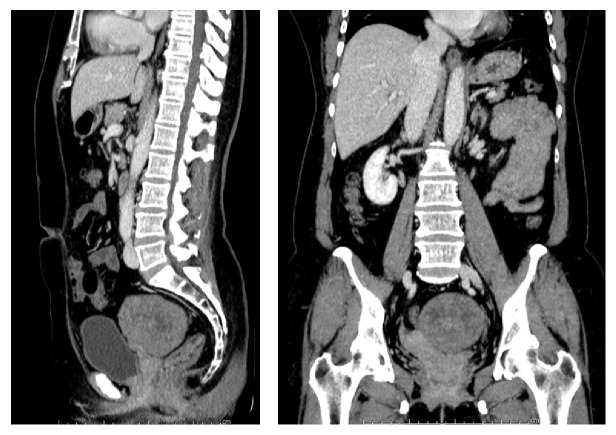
Computed tomography of abdominopelvic region shows heterogeneous enhancing mass in left side of the pelvis 8.2 × 8.0 × 7.0 cm in diameter.
